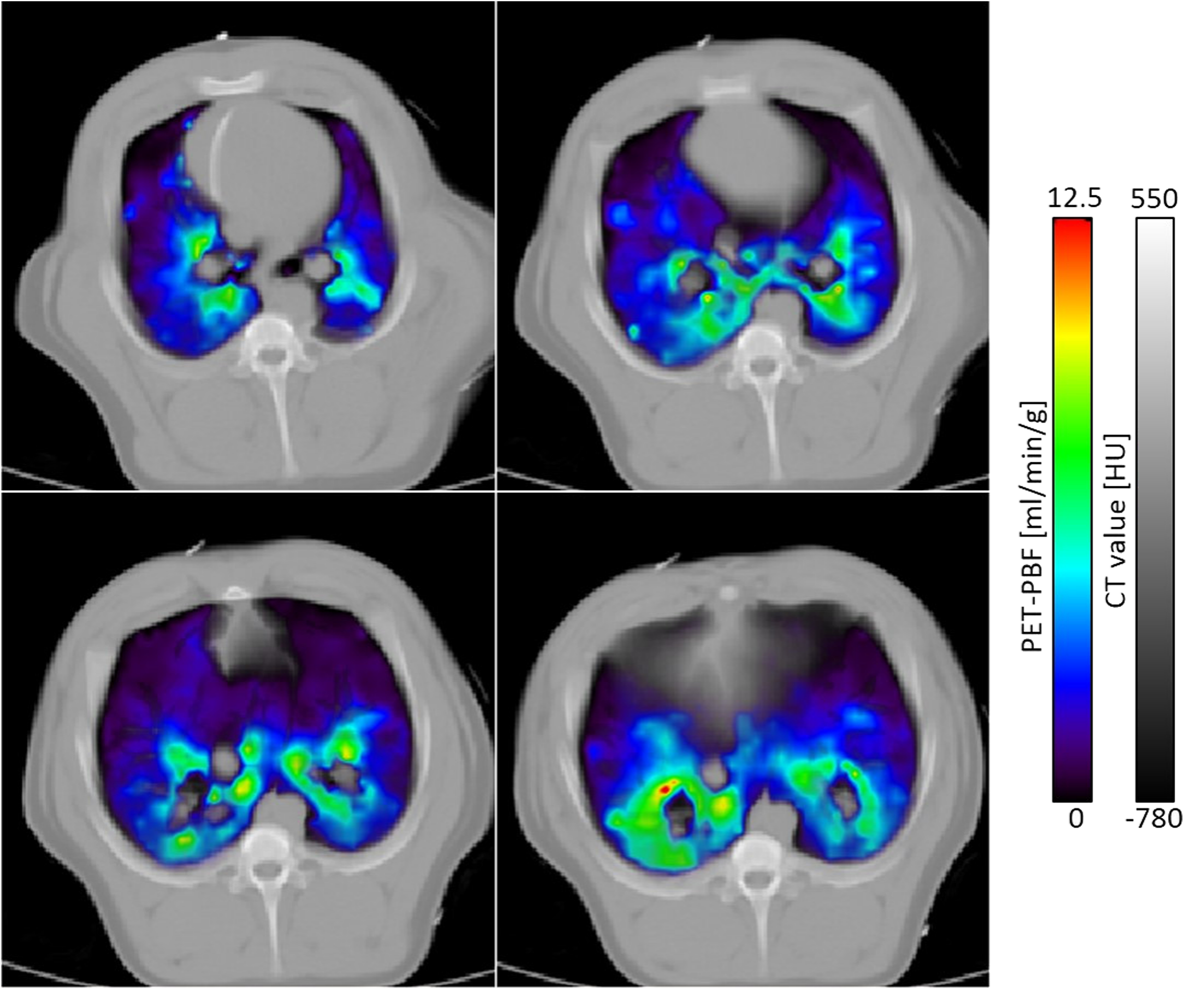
Fused transverse parametric PBF_PET_ and CT images at four different axial locations
